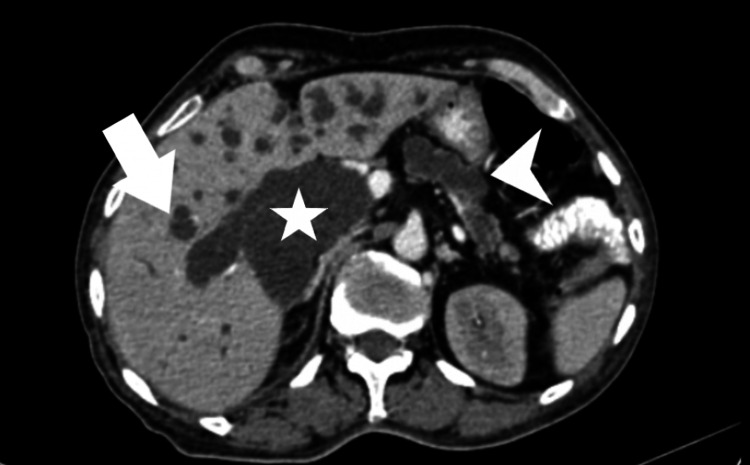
Oral and intravenous contrast-enhanced computed tomographic axial section of the abdomen at the level of the liver showed grossly dilated intrahepatic biliary radicles (arrow), grossly dilated common bile duct (asterisk), and dilated main pancreatic duct (arrowhead).
